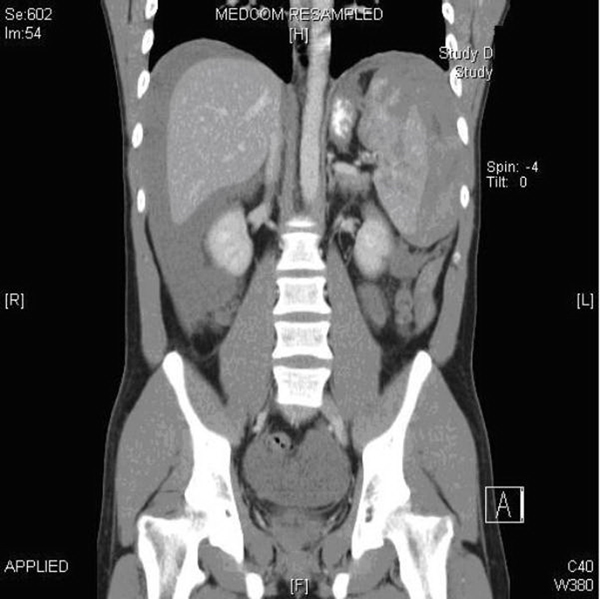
Coronal section on CT Abdomen showing Haemoperitoneum and laceration of the spleen.
